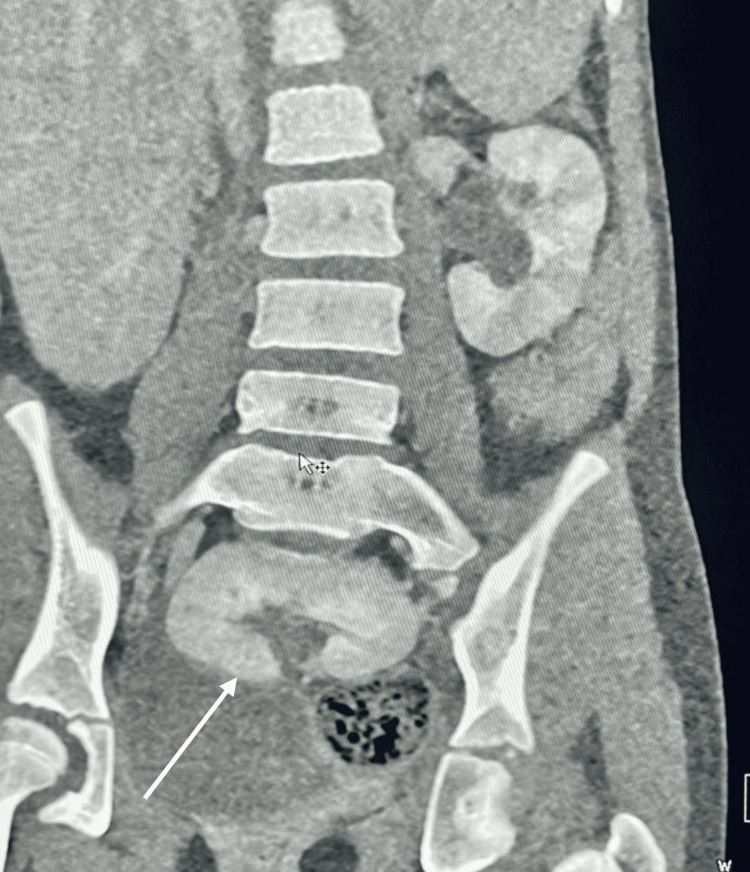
CECT abdomen showing ectopic right pelvic kidney The white arrow points to the ectopic right pelvic kidney. CECT, contrast-enhanced computed tomography.
